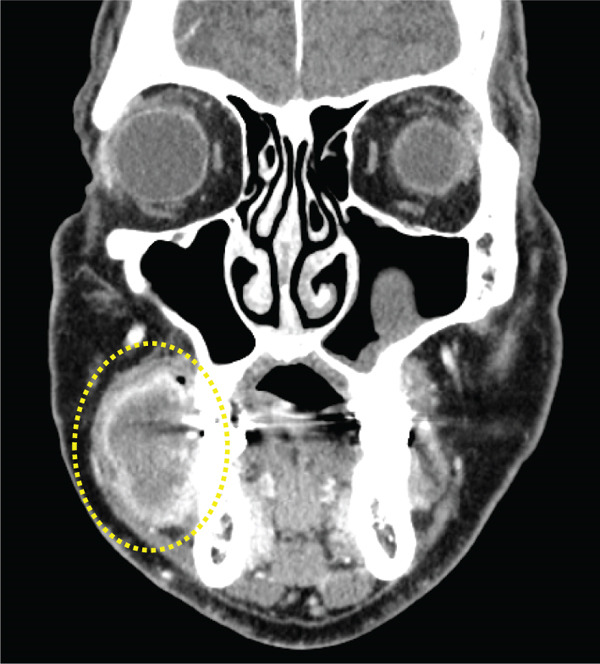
Contrast‐enhanced CT image showing no calcification or bone invasion.
